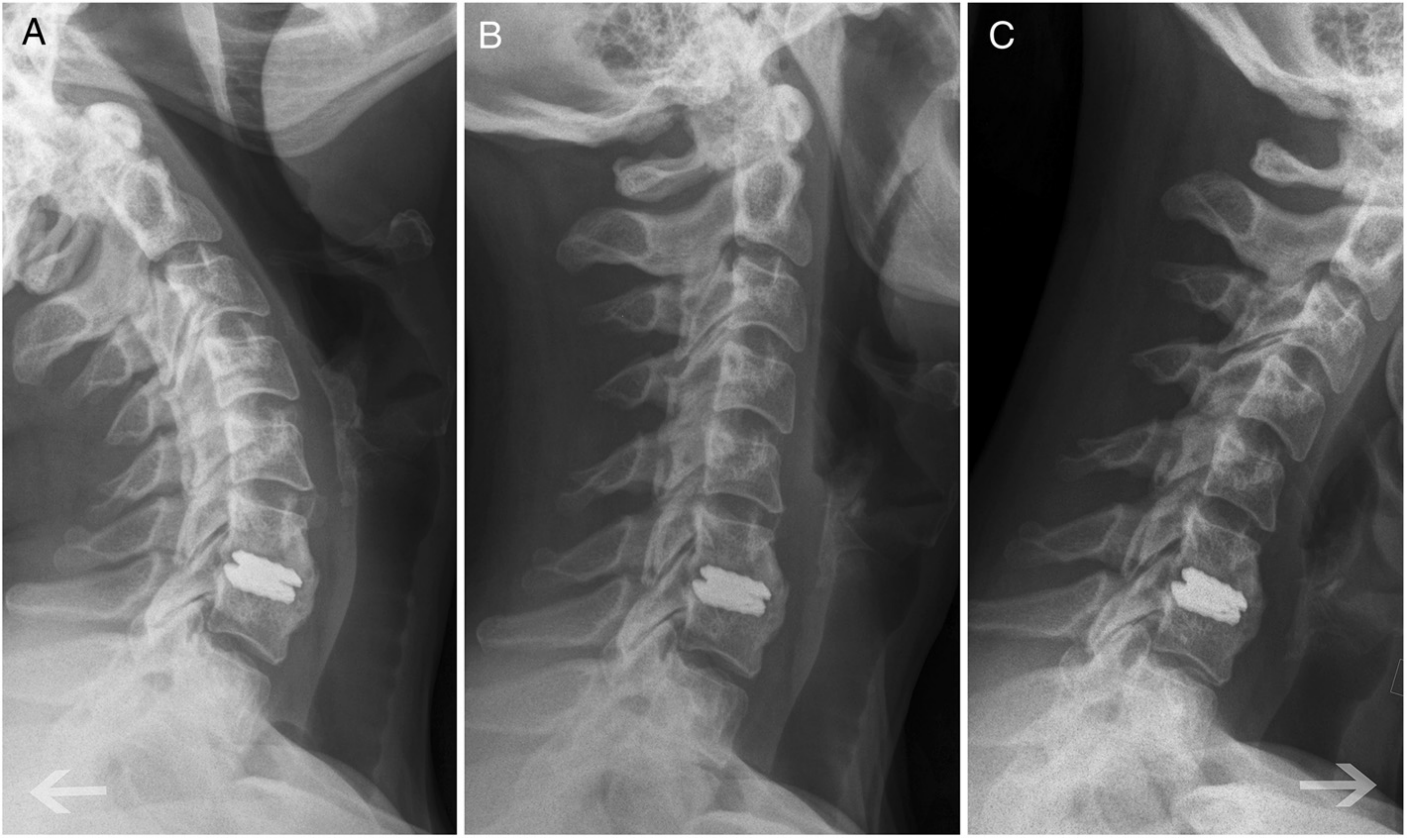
Flexion (a), neutral (b) and extension (c) x-ray showing an immobilized ROTAIO cervical disc prosthesis due to heterotopic ossification at the level C6-C7 in a 48 years old female patient, 12 month after implantation
